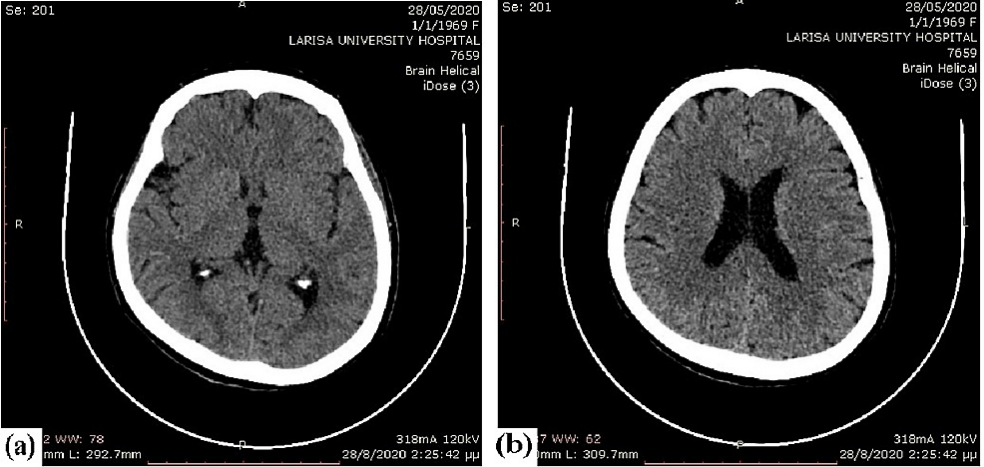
CT brain of the patient revealed no pathological findings.
